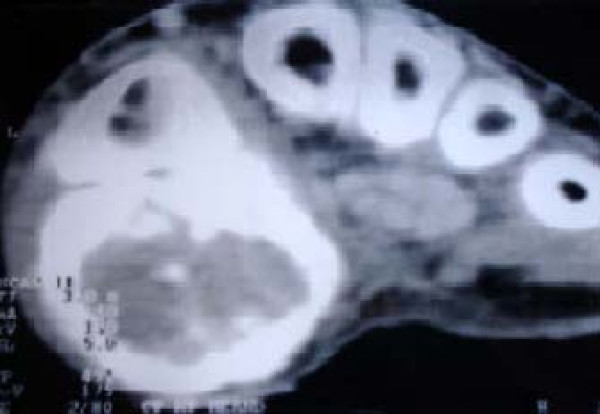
Axial view of hand by CT scan
